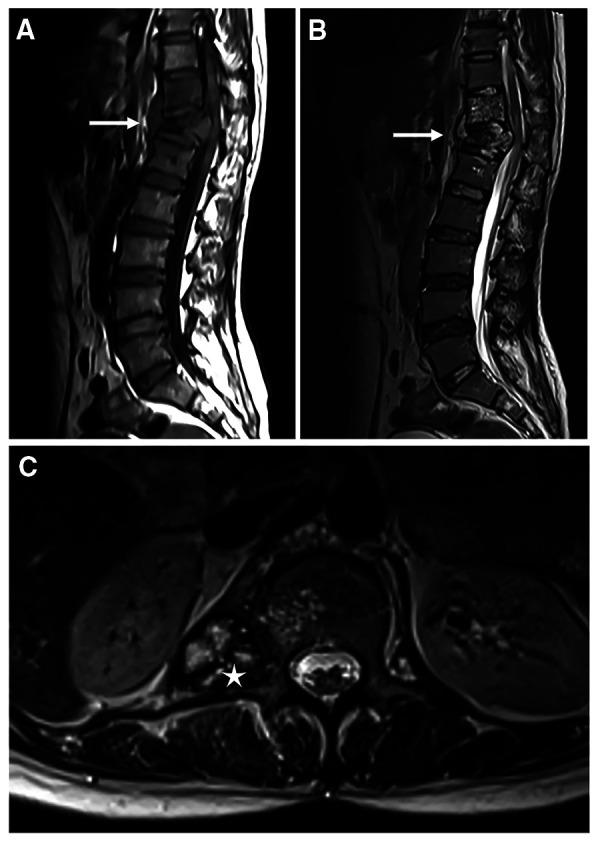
Magnetic resonance imaging of the thoracolumbar spine. T1-weighted image (A) and T2-weighted STIR image (B) of the destructed thoracolumbar spine in sagittal orientation, white arrows indicate destruction of vertebrae; Cystic mass (white asterisk) is found in the T2-weighted image in cross-sectional orientation (C).
